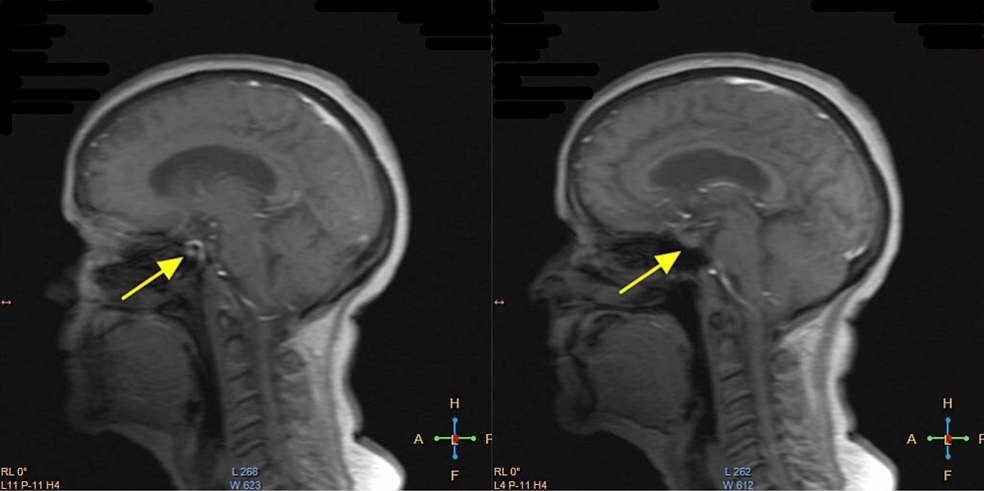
Patient's brain MRI showing pituitary gland enlargement.
